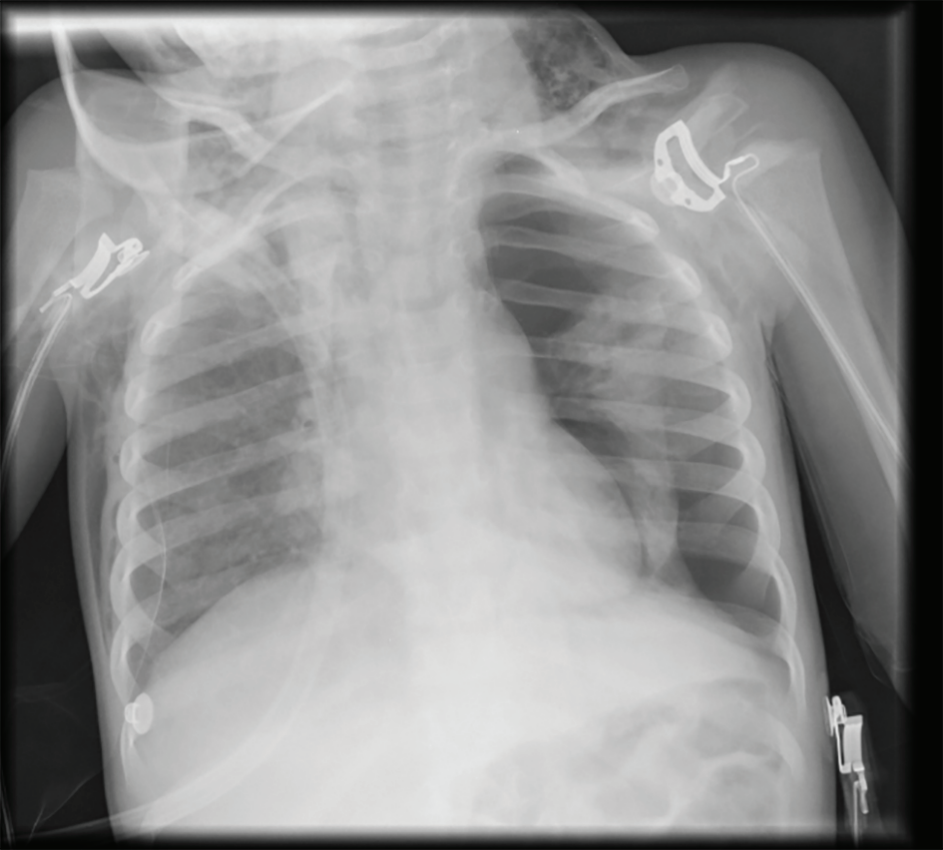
Chest X-ray with left-sided pneumothorax and cervical subcutaneous emphysema.
